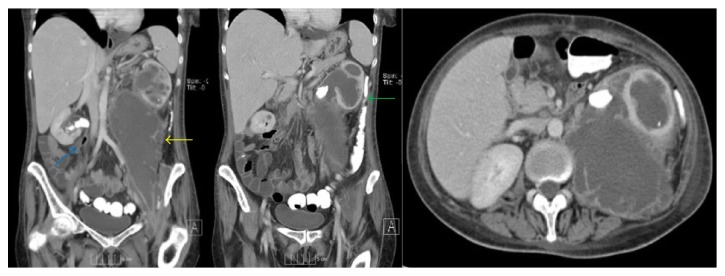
Abdominal CT scan on initial evaluation revealed a large enhancing retroperitoneal fluid collection measuring 11.5 × 22.0 cm (yellow arrow) extending into entire left psoas muscle and hip region. There were bilateral complete duplicated collecting systems with bilateral obstructing large UPJ calculi in both lower pole moieties. The left kidney was remarkable for moderate hydronephrosis of the upper pole moiety and severe cortical thinning of the lower pole. The left kidney was duplicated with moderate upper pole hydronephrosis and moderate-to-severe hydronephrosis with cortical thinning of the lower pole moiety (green arrow). The right kidney had preserved parenchyma with associated emphysematous pyelitis (blue arrow).
